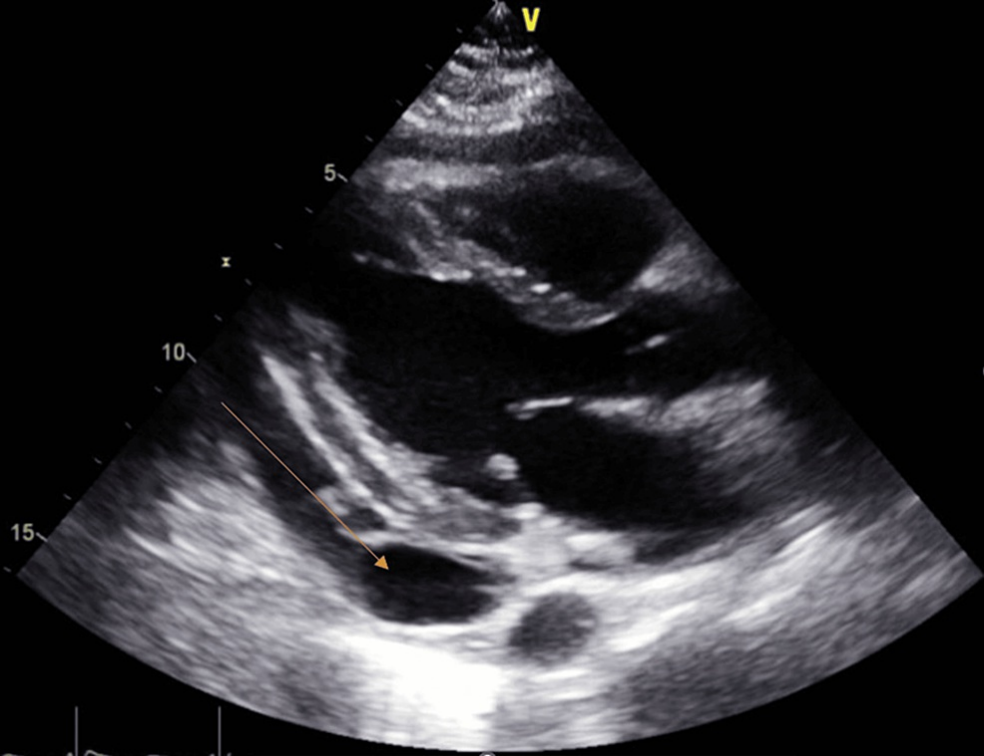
Transthoracic echo on day three showing moderate pleural effusion (orange arrow)
